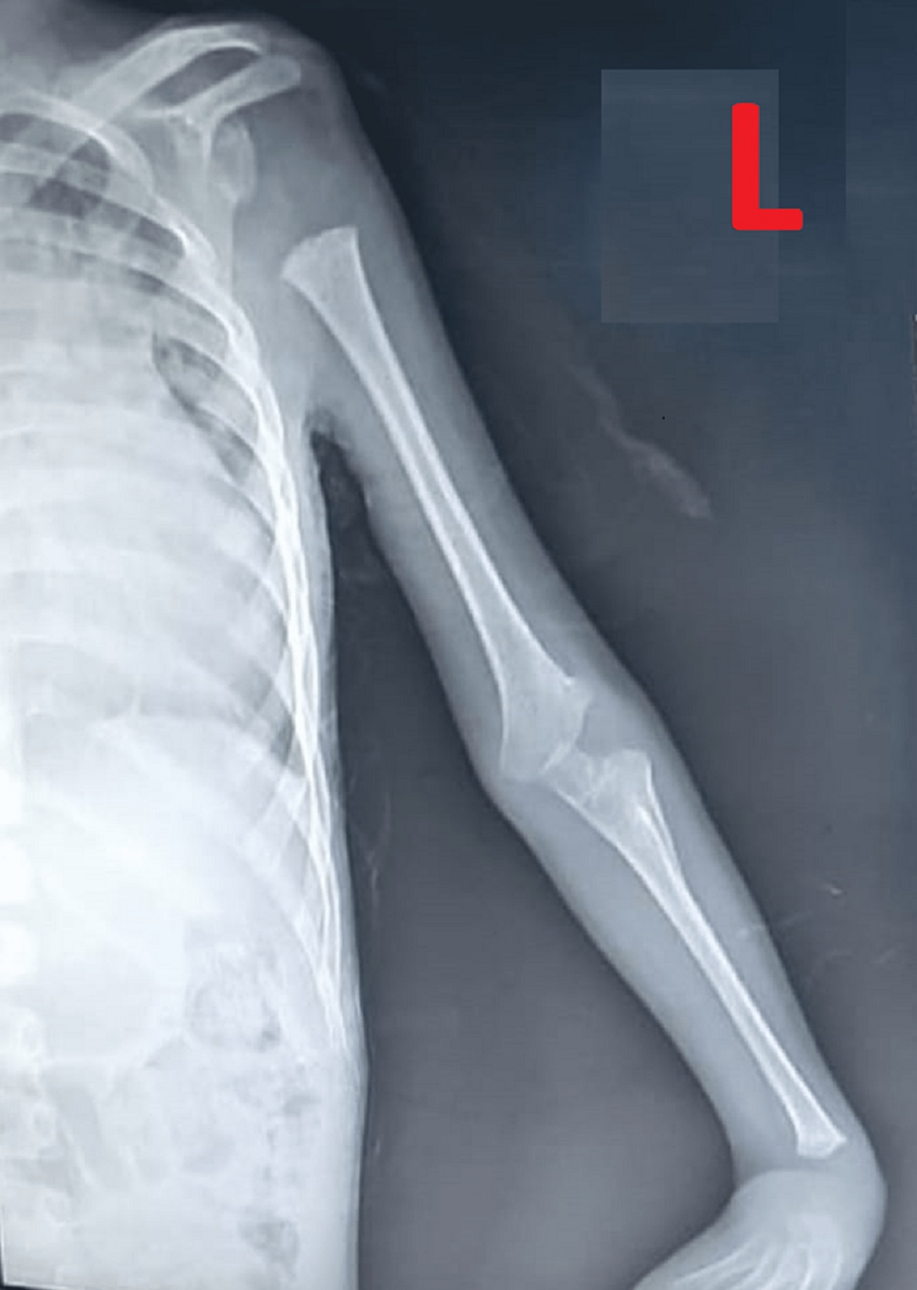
X-ray of Right Limb Showing Skeletal Abnormalities Inform of Hypoplastic Humerus and Absence of Radius With Metacarpal Anomalies
